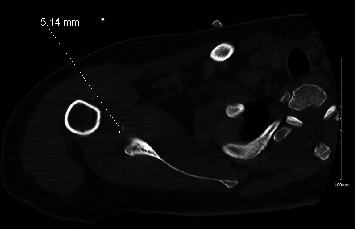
Axial CT scan, right shoulder, postreduction (case 1). The anterior shoulder dislocation has been reduced, and multiple bony fragments measuring up to 5 mm are seen adjacent to the anterior inferior glenoid, representing a bony-Bankart lesion.
